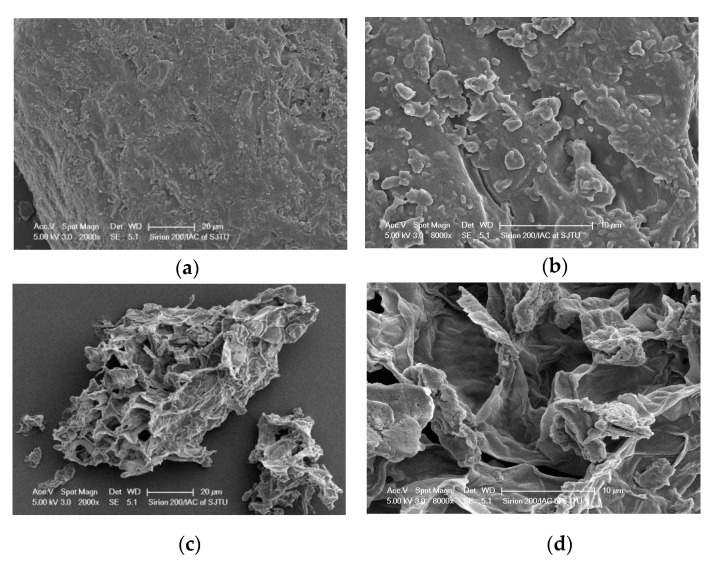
SEM images of the green tea powder before (a,b) and after ultrasonic treatment (c,d). Images (a,c) were taken at 2000× *g* magnification, and (b,d) at 8000× *g* magnification.
